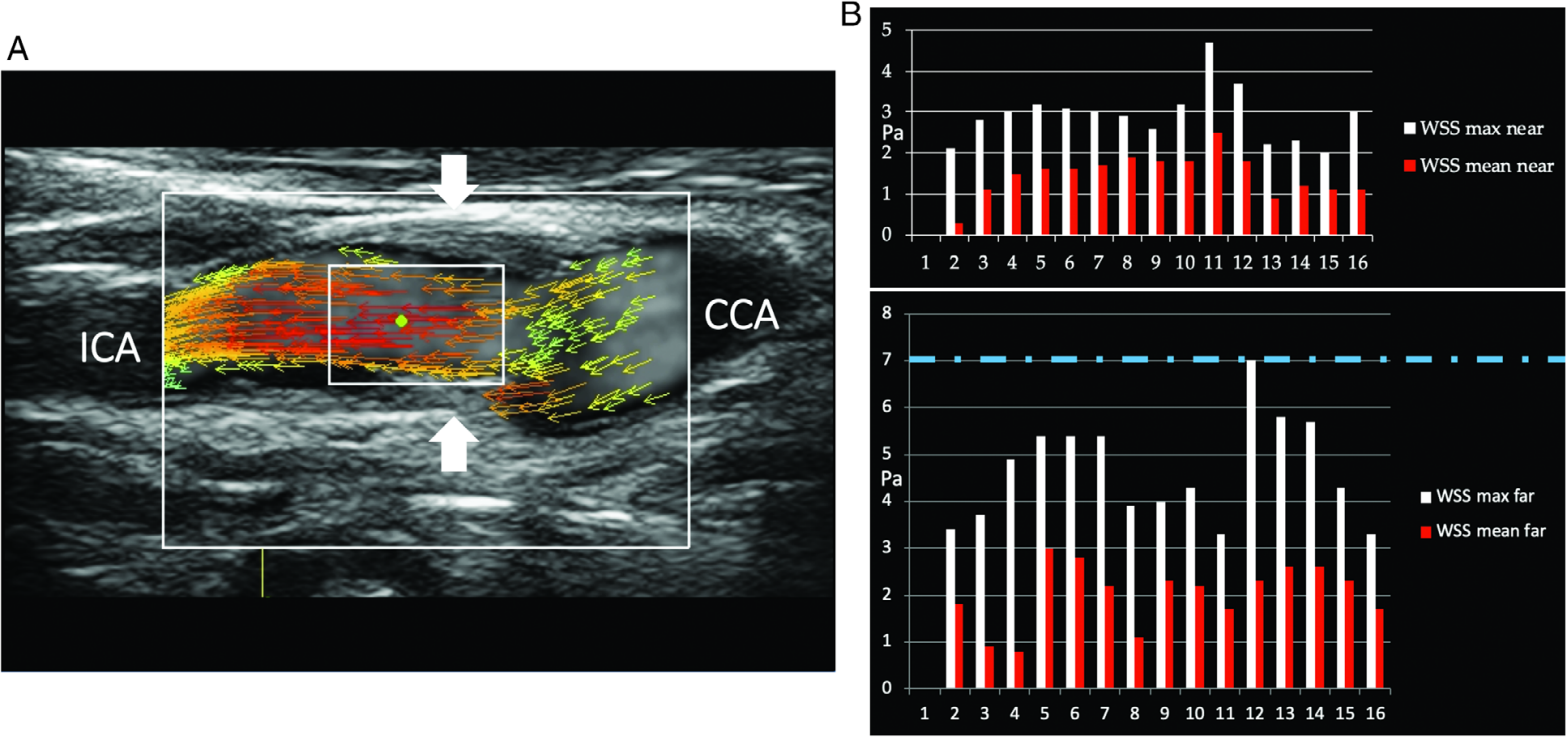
Mild carotid stenosis. A, Ultrasound vector flow imaging shows high‐velocity red vectors moving parallel to the vessel walls inside the stenosis (arrows). B, Wall shear stress point‐by‐point measurements on both the near and far walls had maximum and mean values (2.0–7.0 and 0.8–3.0 Pa, respectively) mainly within the normal range (dashed line corresponds to the maximum normal value). CCA indicates common carotid artery; ECA, external carotid artery; and ICA, internal carotid artery.
